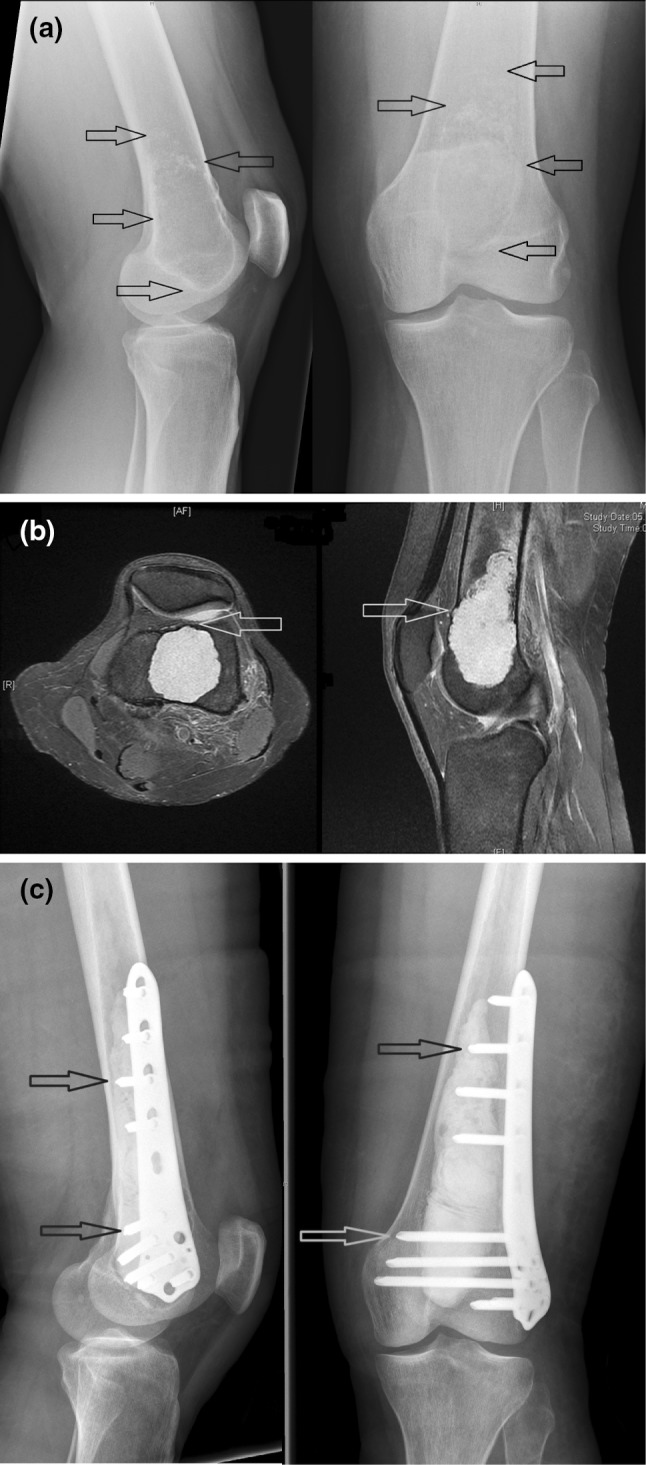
Preoperative X-ray (a) depicts a large atypical cartilaginous tumor (ACT) (black arrows) with typical intralesional popcorn-like ossification in the proximal part (red arrows). Preoperative MRI (b) shows endosteal scalloping and soft tissue extension (red arrows). Postoperative X-ray (c) documents the compound bone cement plate osteosynthesis with integration of the screws of the locking compression plate into the polymethylmethacrylate bone cement (red arrows) to increase stability after intralesional excision of the ACT (color figure online)
